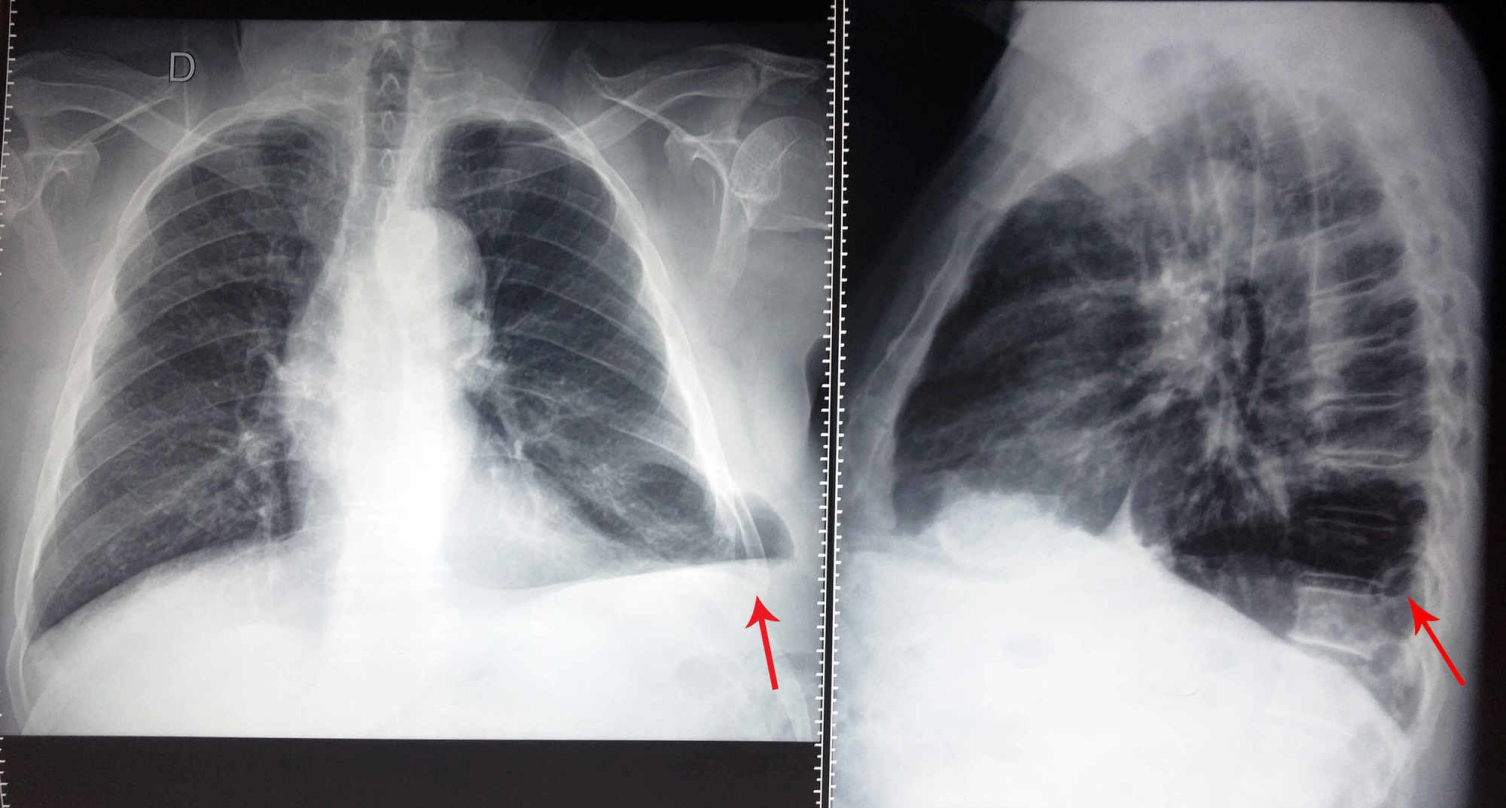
Chest X-ray posteroanterior (A) and lateral (B) view. The red arrow points an oval well-defined hypodensity in the left lower zone.
